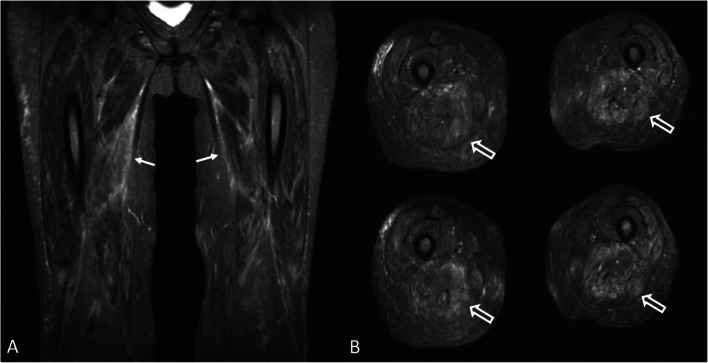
‘Active’ myositis in a SLE patient. Magnetic resonance imaging shows diffuse bilateral edema (arrows) within the adductors muscles on coronal STIR image A. Axial STIR images B show edema and inflammation of posterior thigh muscles (open arrow)
